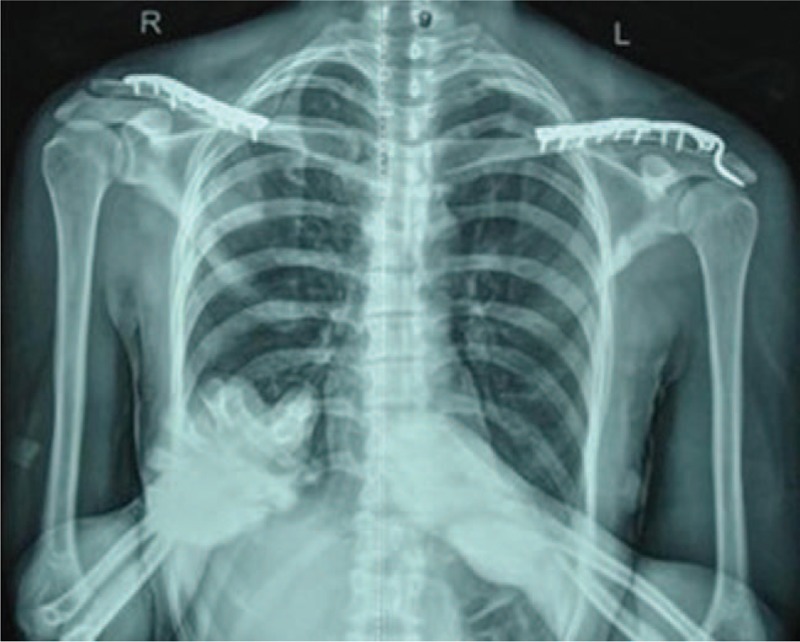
Postoperative radiograph showing the reconstructed clavicle with the truncated anatomic reconstruction plate and the acromioclavicular joint with the hook plate in place.
